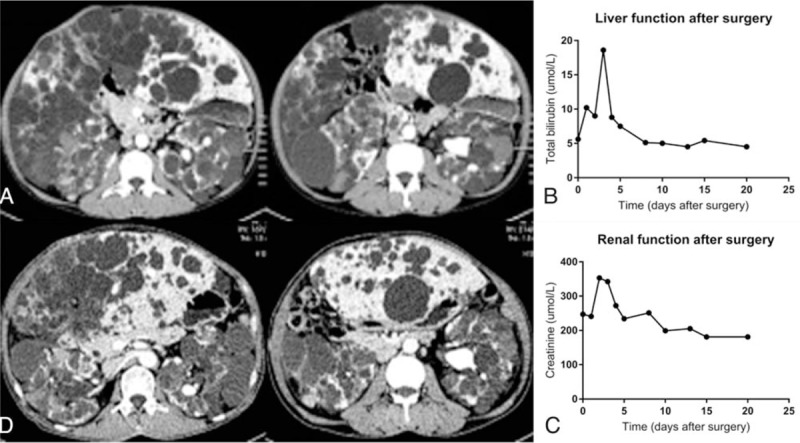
(A) Computed tomographic (CT) scan of the abdomen evidencing enlarged liver and kidneys with multiple cysts and there was few liver parenchyma. (B) Liver function was fully recovered after surgery. (C) Renal function was significantly improved after surgery. (D) Postoperative CT scan of the abdomen showed the growth of liver parenchyma was not as fast as expected (13.8%). CT = computed tomography.
